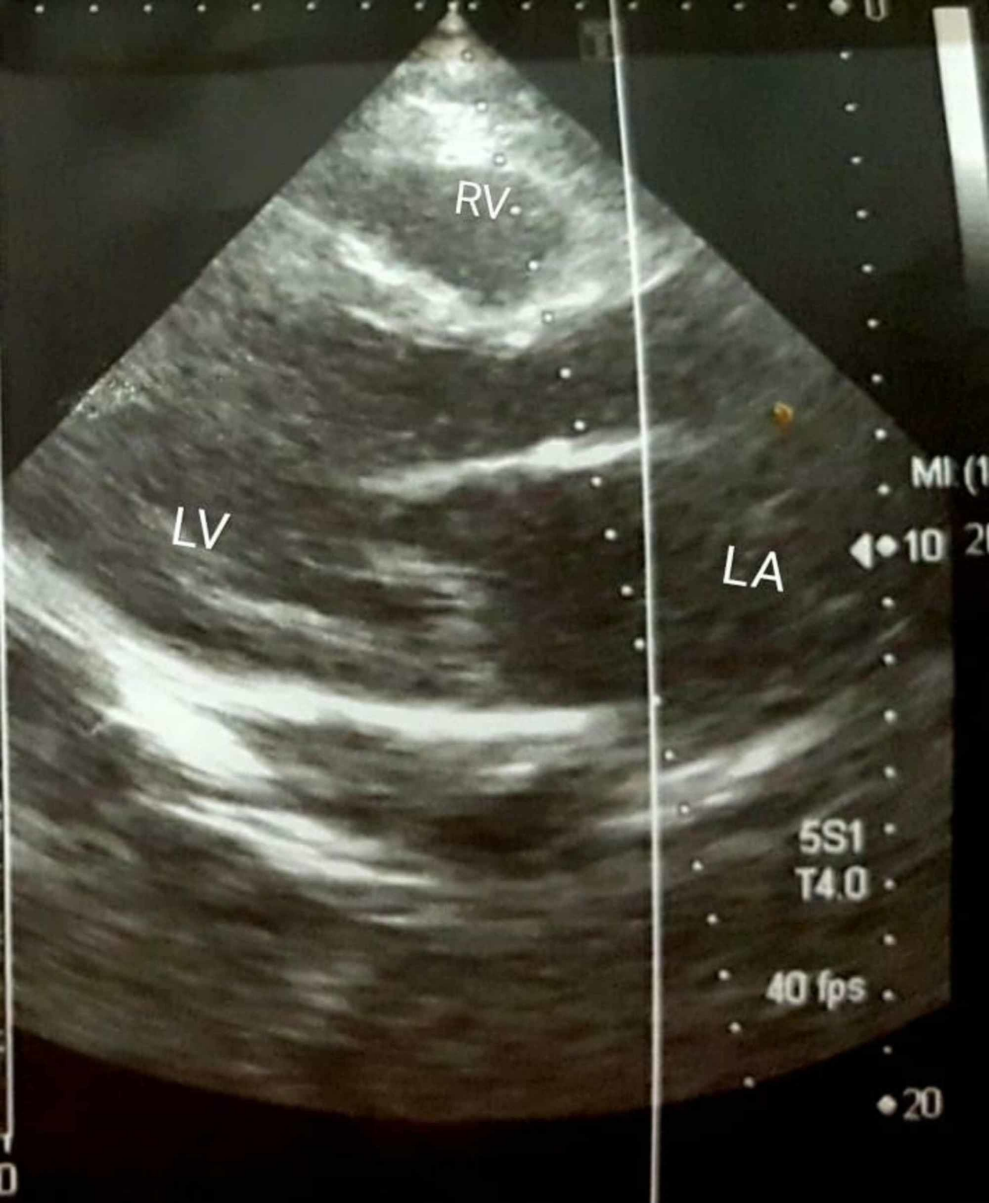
Echocardiogram showing dilatation of LV with wall thinning and mild LA enlargement consistent with dilated cardiomyopathy. LV, left ventricle; LA, Left atrial; RV, right ventricle.
